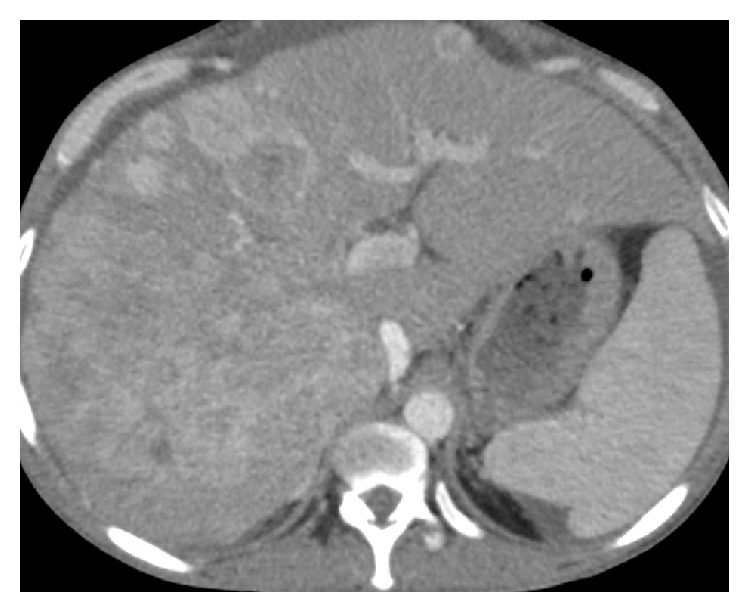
Multiphase abdominal computed tomography: Axial view through the liver showing extensive multifocal HCC.
